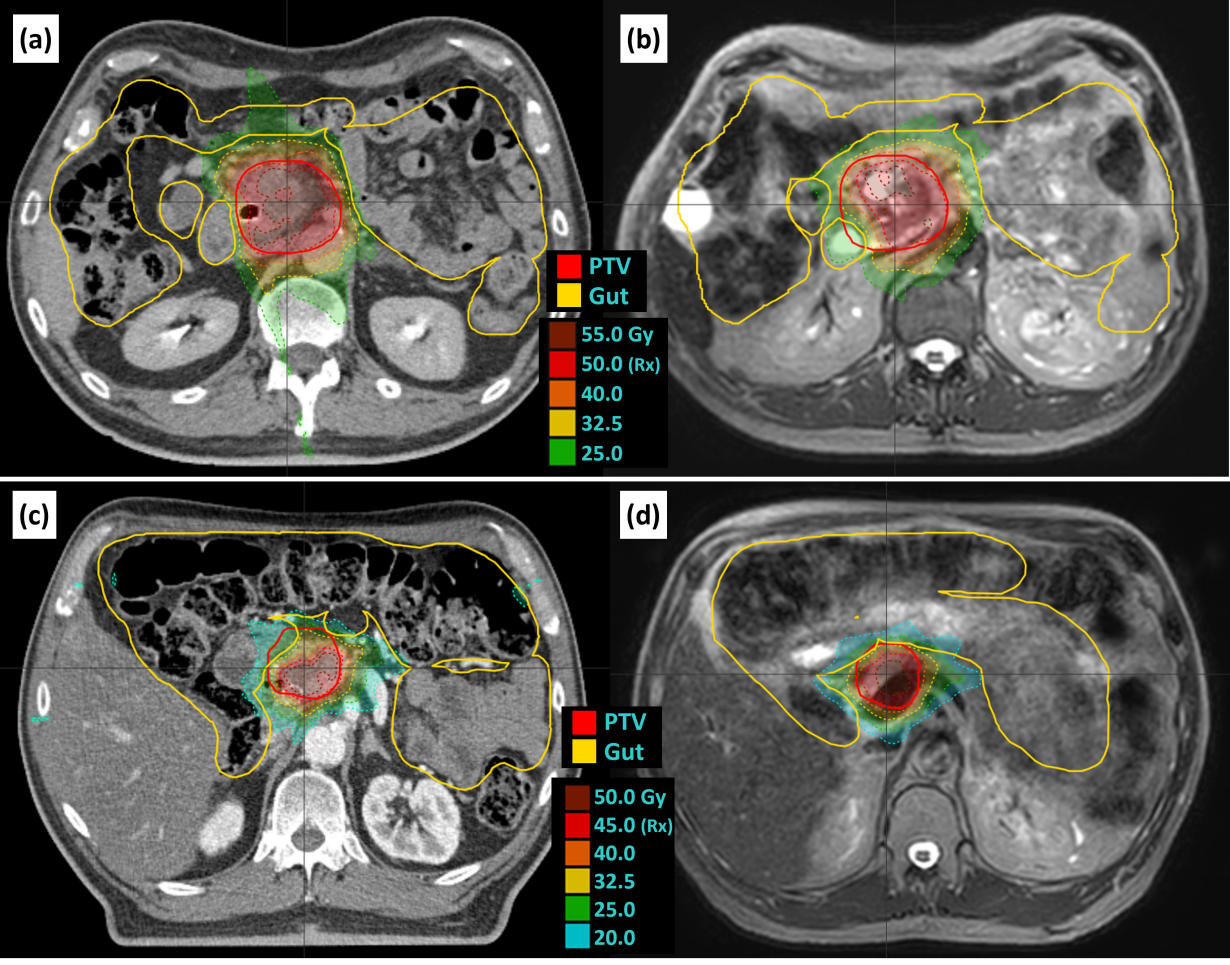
Examples of isotoxic planning. Patient 8 reference plan (A) and fraction 1 (B) as an example of increased overlap and decreased coverage relative to the reference plan. Patient 17 reference plan (C) and fraction 3 (D) as an example of decreased overlap and increased coverage. Panels A and C are reference plans on the reference CT, and panels B and D are example adapted plans on the daily T2 navigator-triggered MRI. CT, computed tomography; MRI, magnetic resonance imaging.
